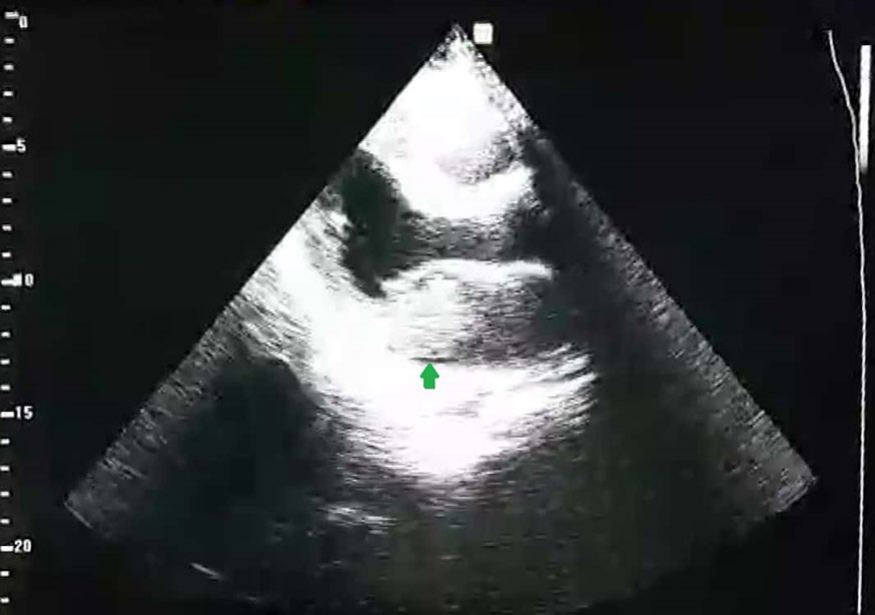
Transthoracic echocardiography showed a large hyperechoic lesion, located in left atrium in the systolic phase (arrow)
